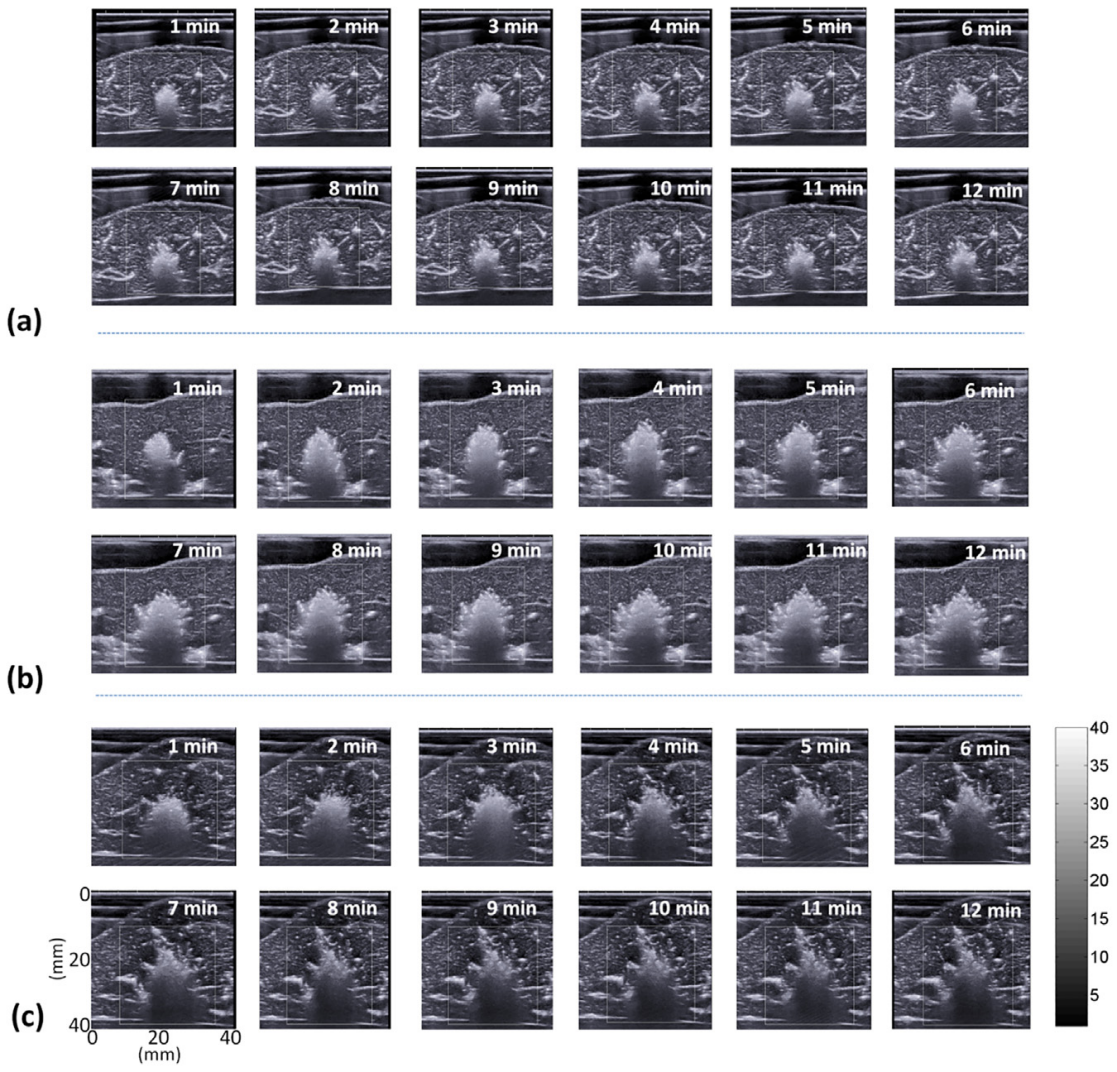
Ultrasound B-mode images acquired at various time points during RFA by using ATLs of (a) 0.5 cm, (b) 1.0 cm, and (c) 1.5 cm.
